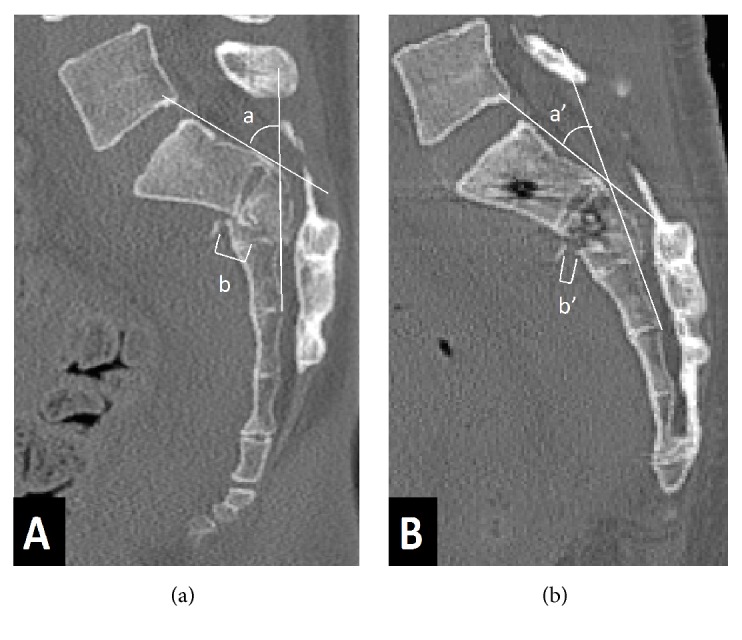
Radiological measurement methods. (a) A preoperative CT scan of the sacrum in the sagittal plane is shown. (b) A postoperative CT scan of the sacrum in the sagittal plane is shown. a and a': sacral kyphotic angle; b and b': sacral fracture translation.
